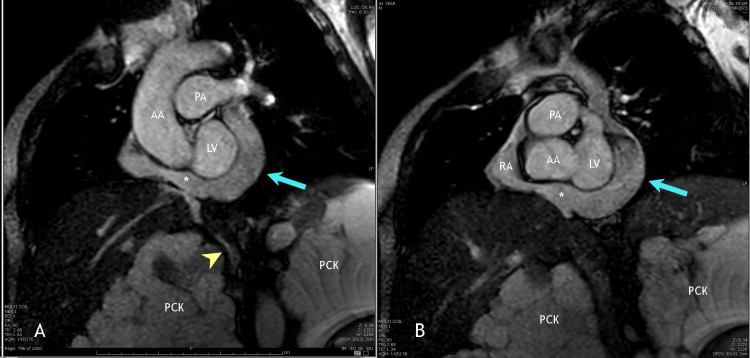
Coronal view of a cardiac MRI study (A) Coronal view of a cardiac MRI study showing the isolated PLSVC (arrow) as it drains into the dilated coronary sinus (*), which then drains into the atrial appendage. Notice the absence of any right-sided superior venous drainage. Also, notice the extremely enlarged polycystic kidneys (PCK), which are compressing the IVC (arrowhead). (B) Coronal view of a cardiac MRI study showing the previously mentioned path of the isolated PLSVC (arrow) into the dilated coronary sinus (*) then into the right atrial appendage (arrowhead). LV: left ventricle, RA: right atrium, AA: ascending aorta, PA: pulmonary artery, PCK: polycystic kidney
